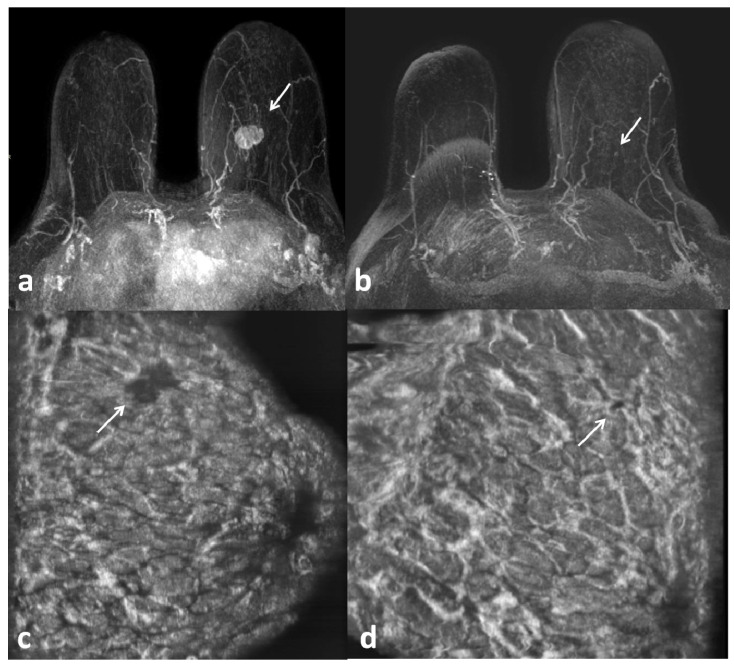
Axial MRI with maximum intensity projection (MIP) reconstructions (a,b) and ABUS coronal images (c,d) taken before (a,c) and after (b,d) NAC. HER2+ breast cancer of the left breast with complete response to NAC (arrows).
